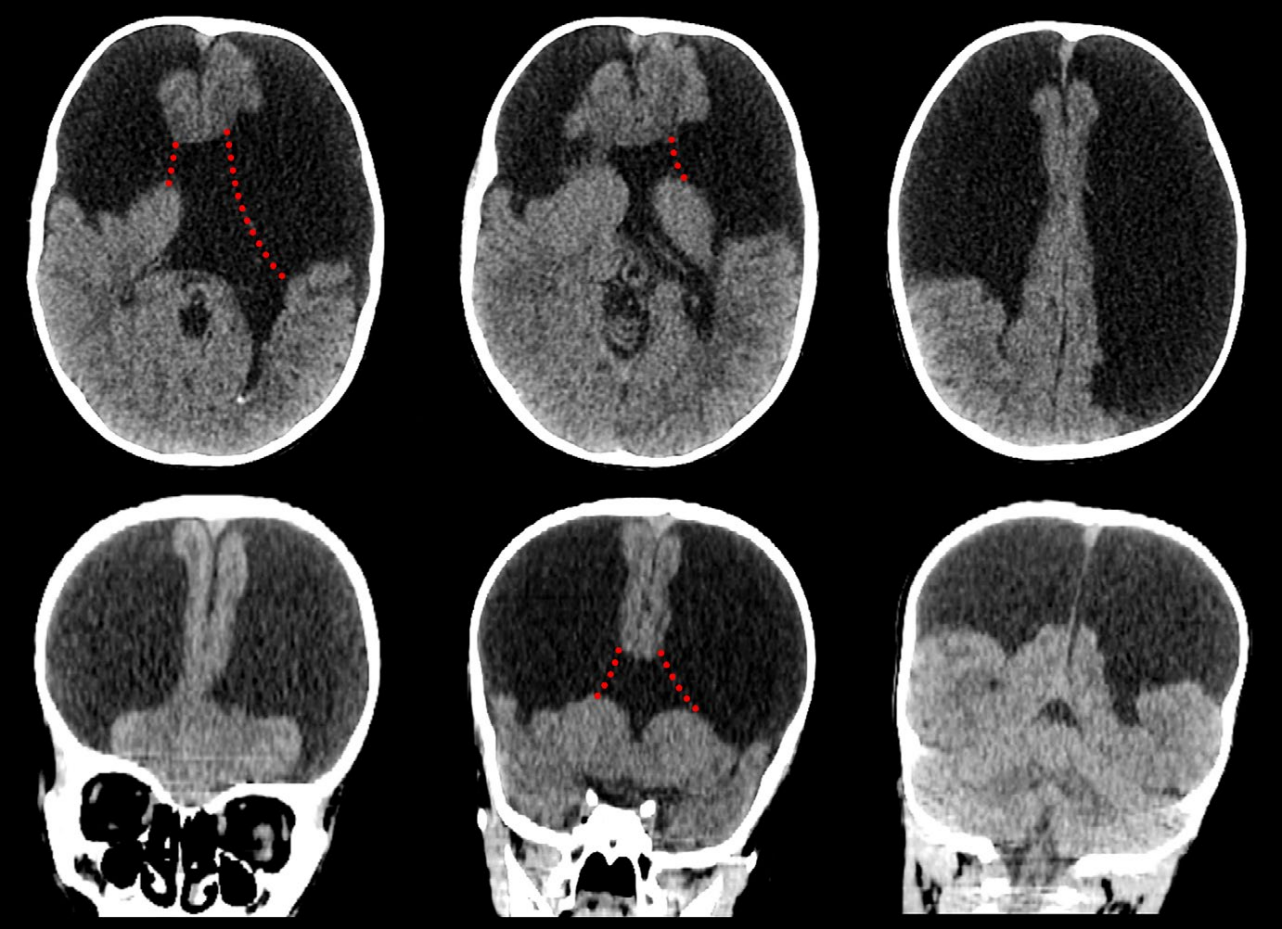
CT images show large brain defects in most of the bilateral frontal, parietal, and temporal lobes communicating with the ventricles by wide clefts (red dotted lines). The septum pellucidum and corpus callosum are absent. Note that MRI is more sensitive than CT for detecting gray matter abnormalities
